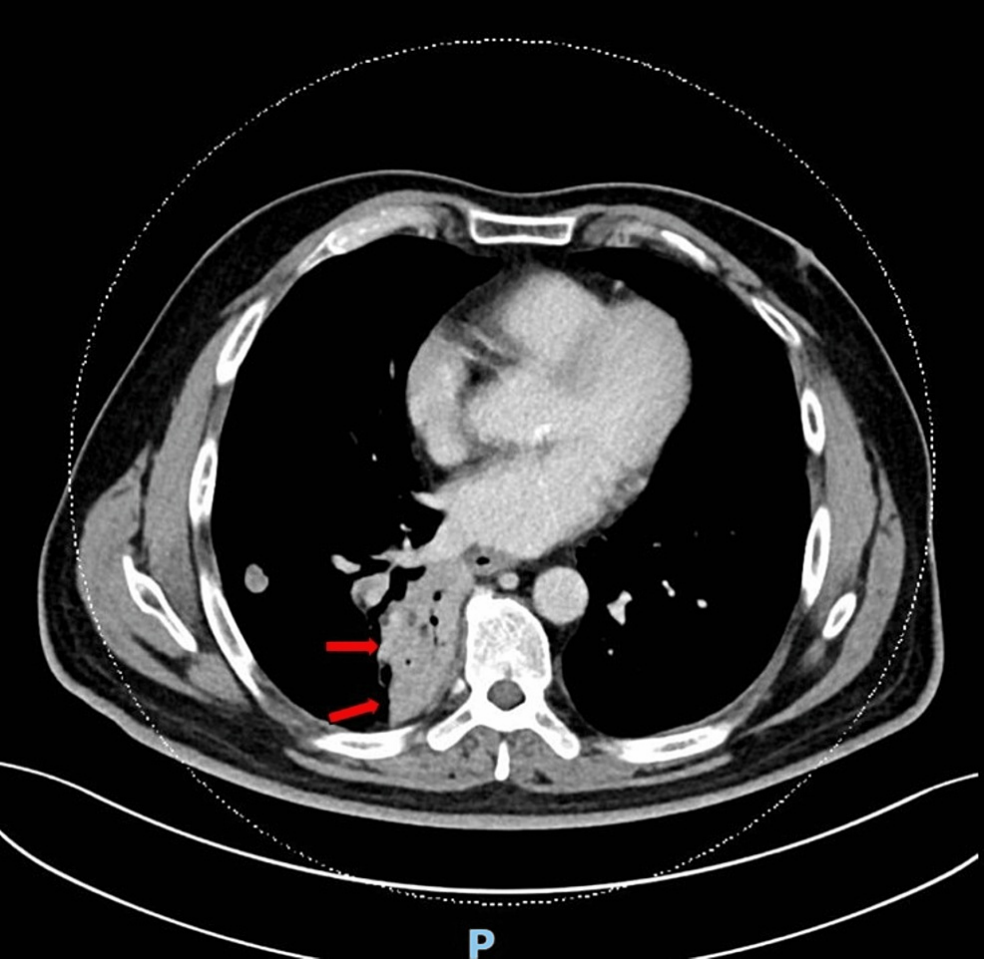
Computed tomography of the thorax axial view with new mass-like consolidation in the right lower lobe.
